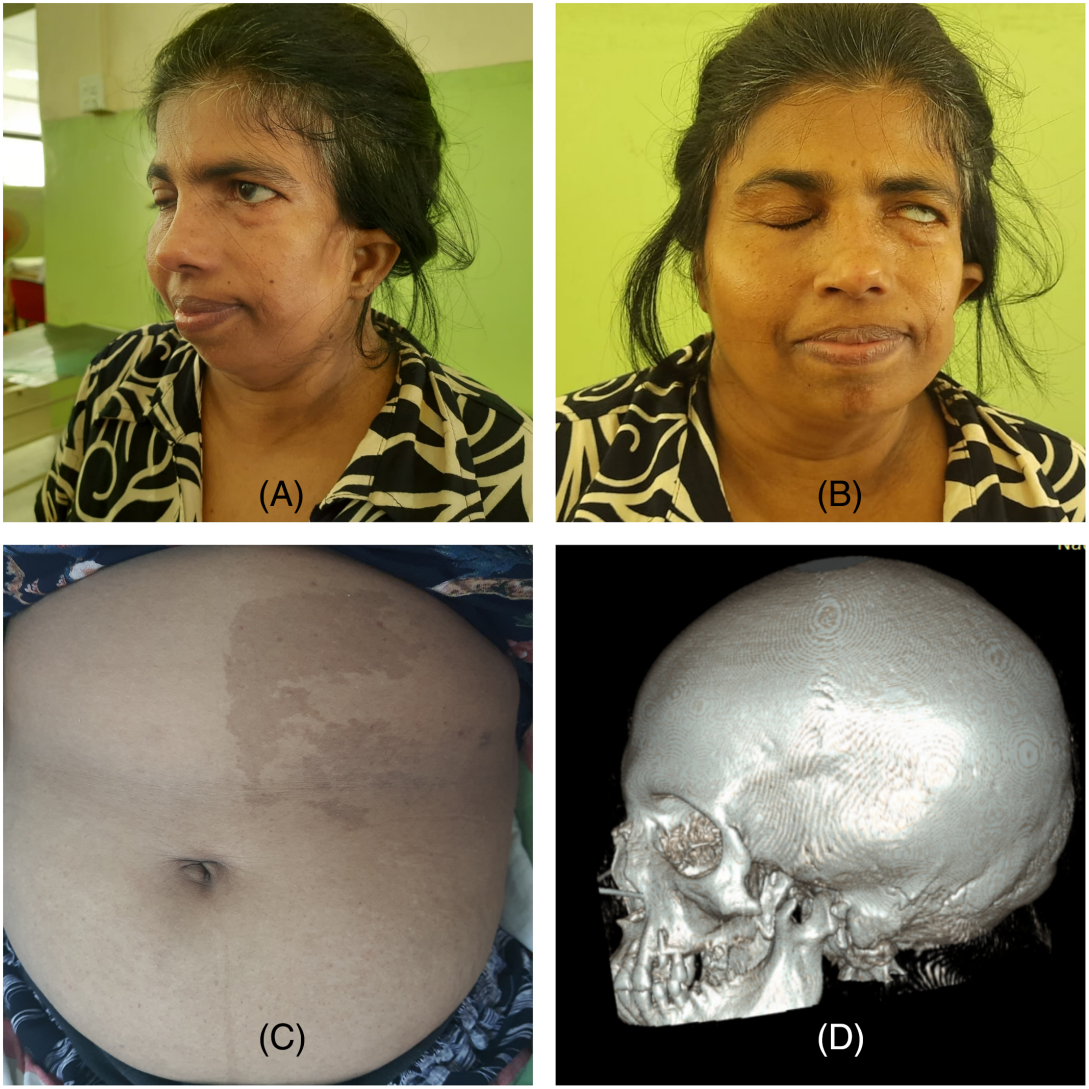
(A, B) Left hemi facial muscle atrophy, microtia (C) café au lait patch in anterior abdominal wall, (D) absent left zygomatic arch in lateral view of skull CT construction.
